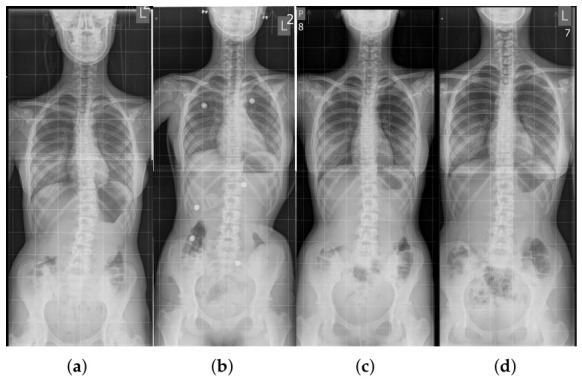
X-ray image of the spine of the first patient: (a) At the start of treatment. (b) While wearing the orthopedic brace. (c) During a follow-up visit in the course of therapy. (d) Without the brace, correction maintained.
